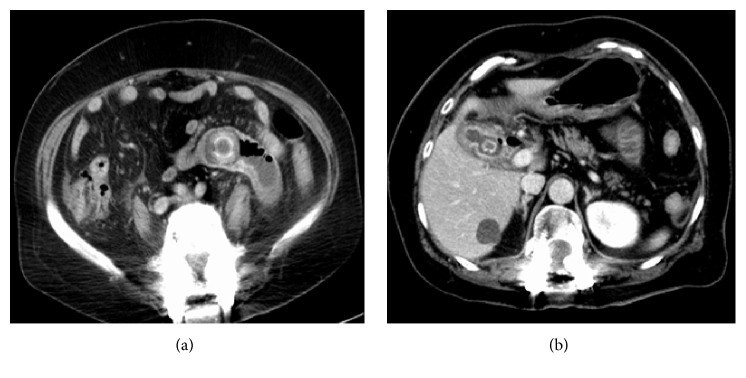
Computed tomography (CT) images showing (a) small bowel obstruction by a partially calcified object in the jejunum and (b) inflammation around the gallbladder, with a gallstone, and thickening between the gallbladder and the duodenal walls. Air-fluid levels in the gallbladder were also seen.
